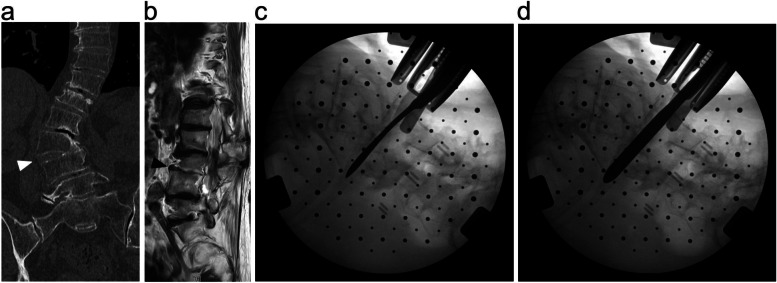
a This degenerative lumbar kyphoscoliosis case had fused vertebrae at L3–4 (white arrowhead). Anterior vertebral autofusion was found in the concave side of the lumbar curve. b In the same case, the remnant disc was found on T2 weighted image of magnetic resonance image at the intervertebral space of L3/4 level (black arrowhead). c A Cobb elevator with 18 mm width was inserted into the disc space from the non-fused side and penetrated to the contralateral side under a fluoroscope. d Subsequently, a 6 mm thick Paddle Sizer® was inserted to completely accomplish the release between the fused vertebrae
